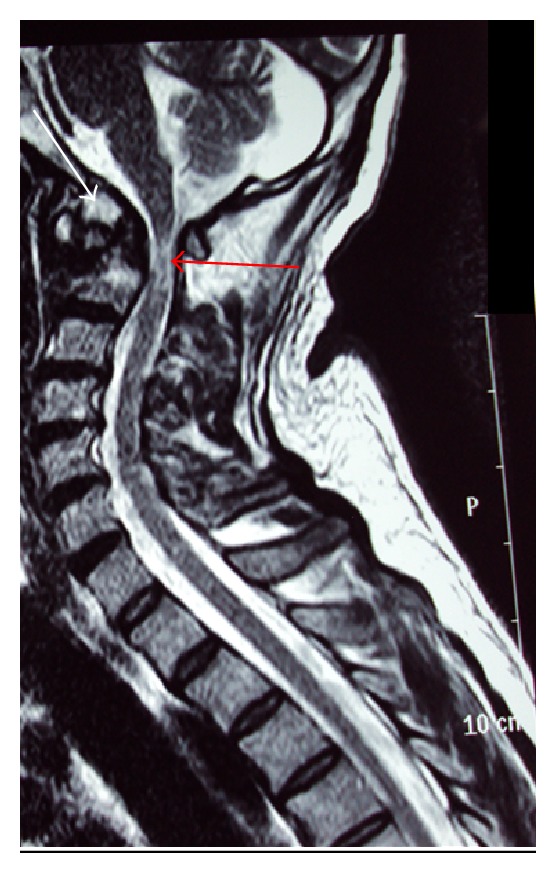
MRI in T2 sequence of the cervical spine. Red arrow: chronic myelopathy (hyperintense signal) can be seen. Anterior to that the bone mass (pseudarthrosis) is seen. The white arrow is indicating the odontoid process of the axis (C2 vertebrae).
